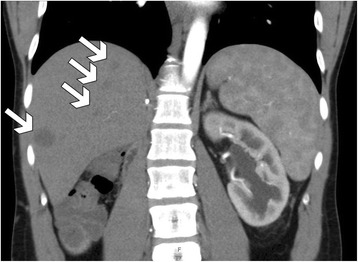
Abdominal CT scan at 3 months postoperatively-multiple liver metastases (*white arrows*)
